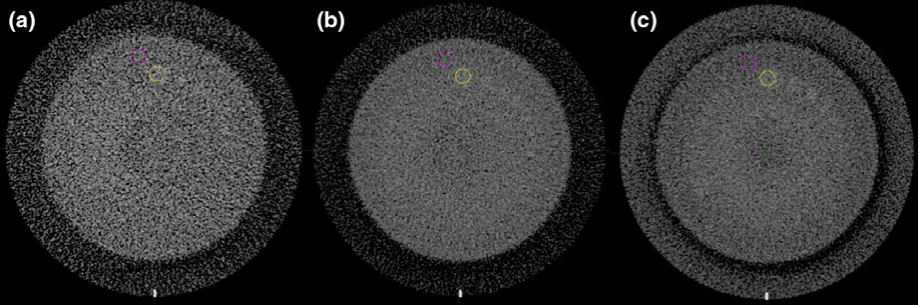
Comparison of the Catphan low contrast module scanned with different CBCT protocols. (a) standard half‐rotation 100 kVp 15 mA scan (wCBDI — 0.3 cGy); (b) full‐rotation 100 kVp 30 mA scan (wCBDI — 1.2 cGy); (c) full‐rotation 80 kVp 70 mA scan (wCBDI — 1.2 cGy). HU window: [0, 300 HU]. Two contours are for contrast‐to‐noise ratio measurements.
